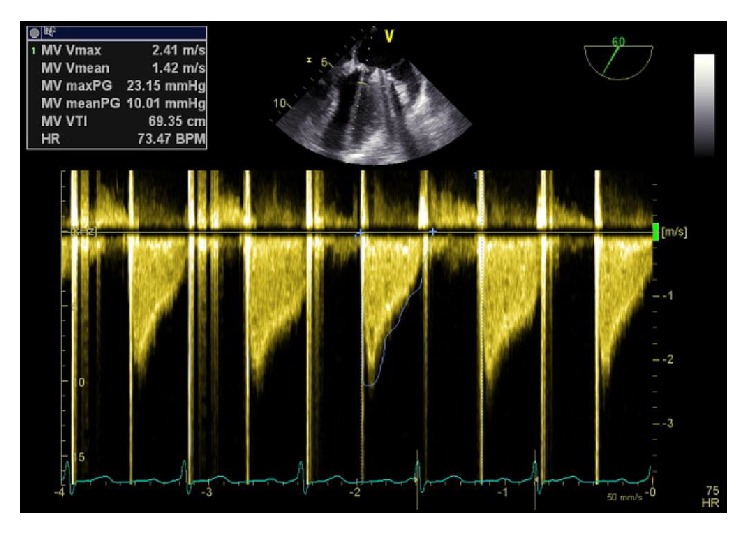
Transesophageal echocardiography of a mitral valve prosthesis. CW-Doppler recording of the transmitral flow shows an increased transvalvular gradient.
